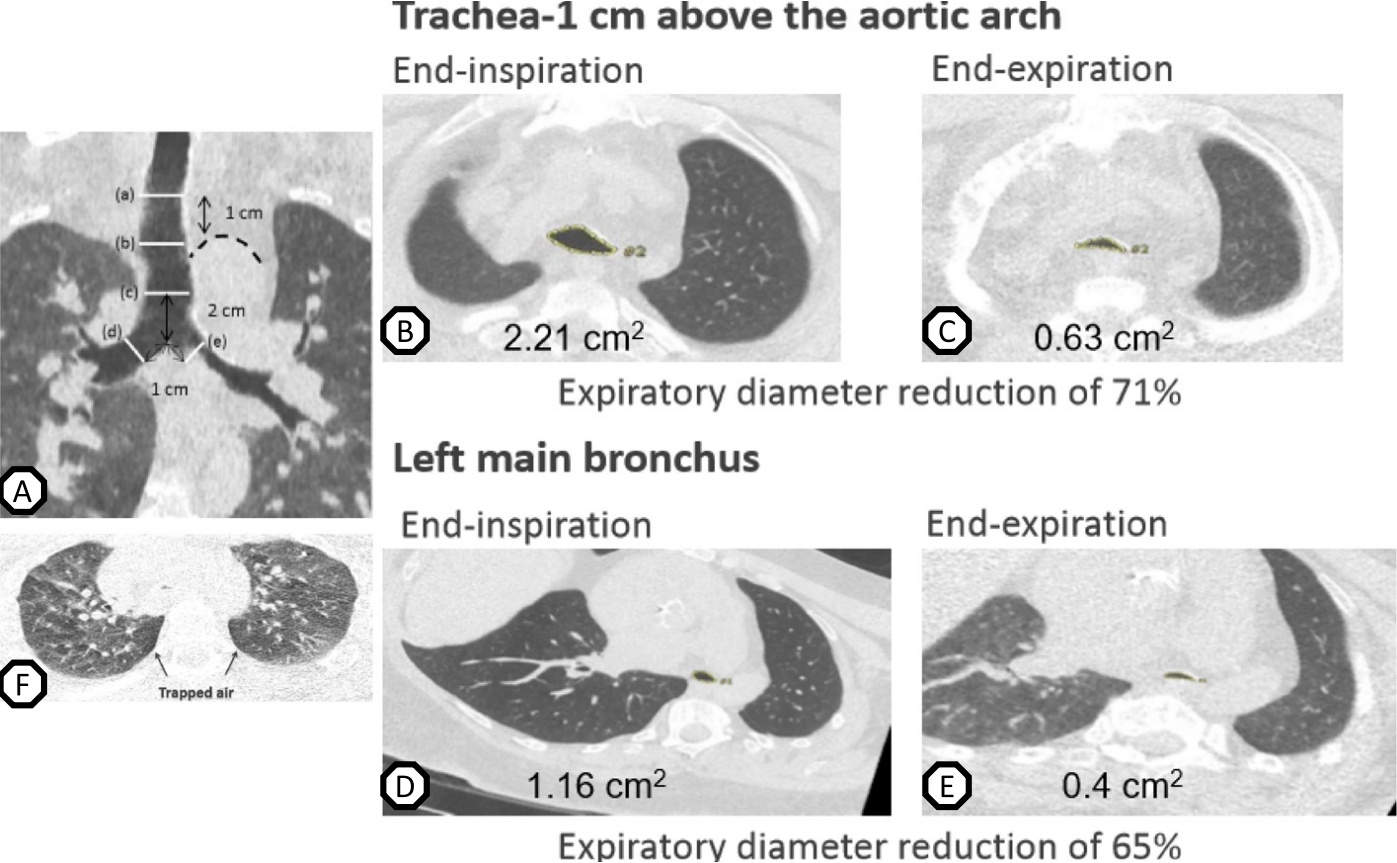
In- and expiratory chest CT scan. a Central airways measurements were performed at five locations: (a) 1 cm above aortic arch, (b) aortic arch, (c) 2 cm above carina bifurcation, (d) right main bronchus, (e) left main bronchus. b Tracheal cross section at “b” (1 cm above the aortic arch) in patient 2. Note abnormal “bell-shaped” trachea in the end-inspiration image. c At end expiration there is almost complete collapse of the trachea (same patient). d Bronchial cross section at “e”(left main bronchus) at end inspiration (patient 2) and e at end expiration (same patient). f Presence of trapped air at expiration (patient 1)
